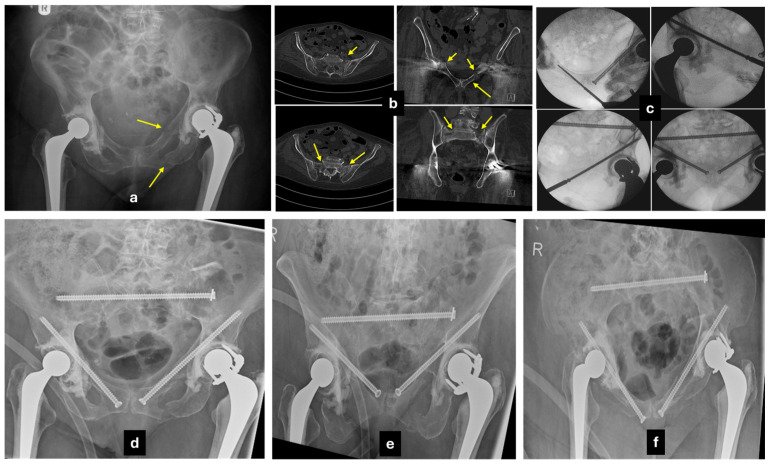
Case example of percutaneous fixation of both the anterior and posterior pelvic ring following an FFP-IIIC. This was a female patient, 86 years old, with a medical history of chronic kidney disease, atrial fibrillation, and bladder cancer, who presented following a simple fall. (a) Anteroposterior plain X-ray with left-sided superior and inferior rami fractures and suspicion of left sacral fracture. (b) Slices of CT-scan with clear identification of fracture planes (yellow arrows). The fracture was classified as FFP-IIIc. (c) Fluoroscopic views taken during the fixation of this FFP with two retropubic cannulated position screws, 6.5 mm, and one 8.0 mm transiliac-transsacral fully threaded cannulated screw, at the S1 level. The patient was mobilised without limitations postoperatively using a Zimmer frame for 6weeks. (d) Anteroposterior; (e) outlet; (f) inlet X-rays taken 13 months later with union and restoration of patient pre-injury mobility.
